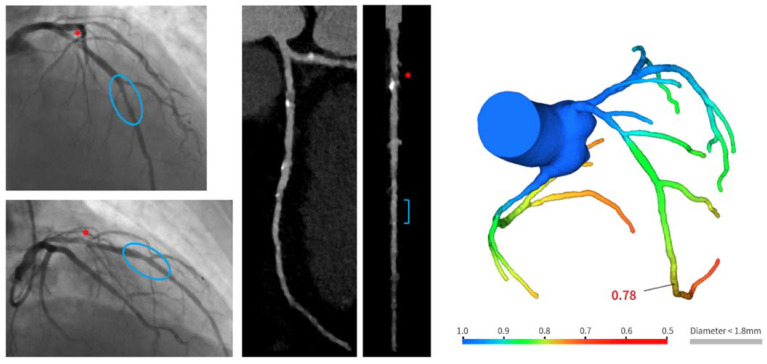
Example of positive CT-FFR on target vessel. Angiographic images show culprit stenosis on the mid LAD (upper image), treated with one RMS (Magmaris^®^ 3 × 25 mm) (lower image). In the angiographic images, the red asterisk indicates an intermediate stenosis in the proximal LAD segment. Follow-up CCTA highlighted the long-term patency of the segment treated with RMS (bracket), showing the progression of the disease in the proximal portion (red asterisk), with the positive presence of prevalent soft plaque at CT-FFR assessment (value 0.78).
